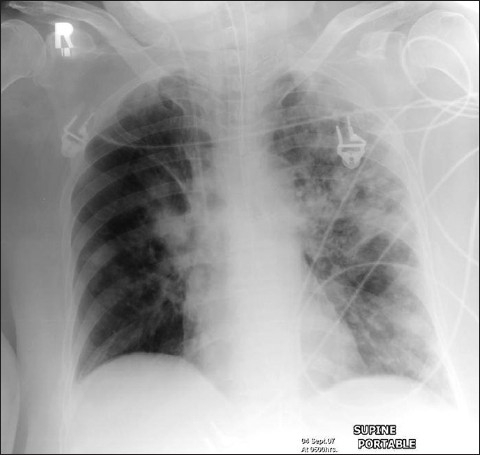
An AP supine radiograph on an intubated patient showing patchy consolidation in both lung fields, more prominent on the left due to hospital-acquired pneumonia
